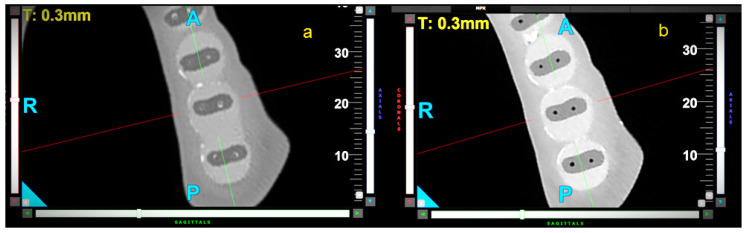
Representative CBCT axial—horizontal slices of samples at 6 mm from apex, before (a) and after (b) canal preparation—reciprocal movement. The letters A, P, R indicate sides—anterior, posterior, and right respectively.
